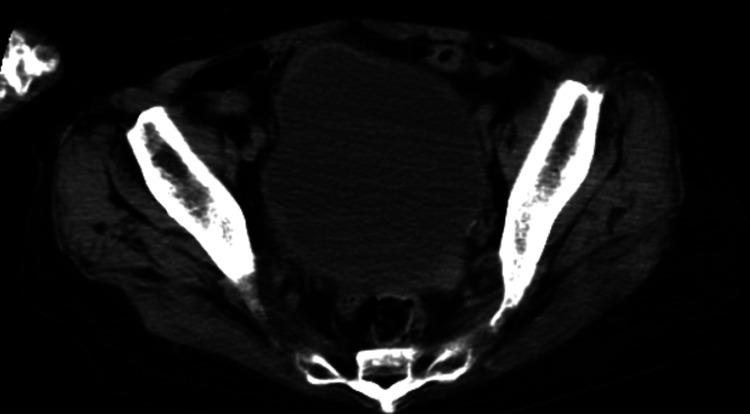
Plain CT of the lower abdomen The plain CT showed bladder distention.
